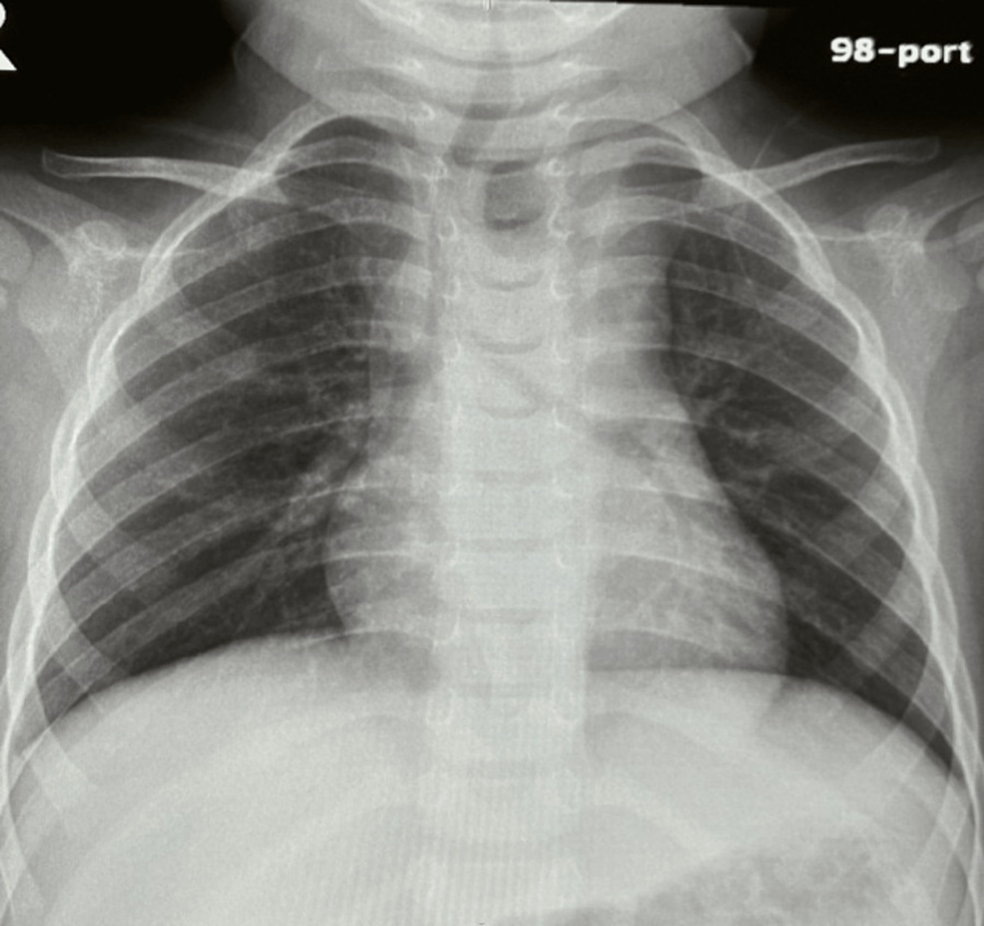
Chest X-ray showing a rounded lucency in the upper esophagus, pushing the trachea to the opposite side with the widening of the mediastinum
